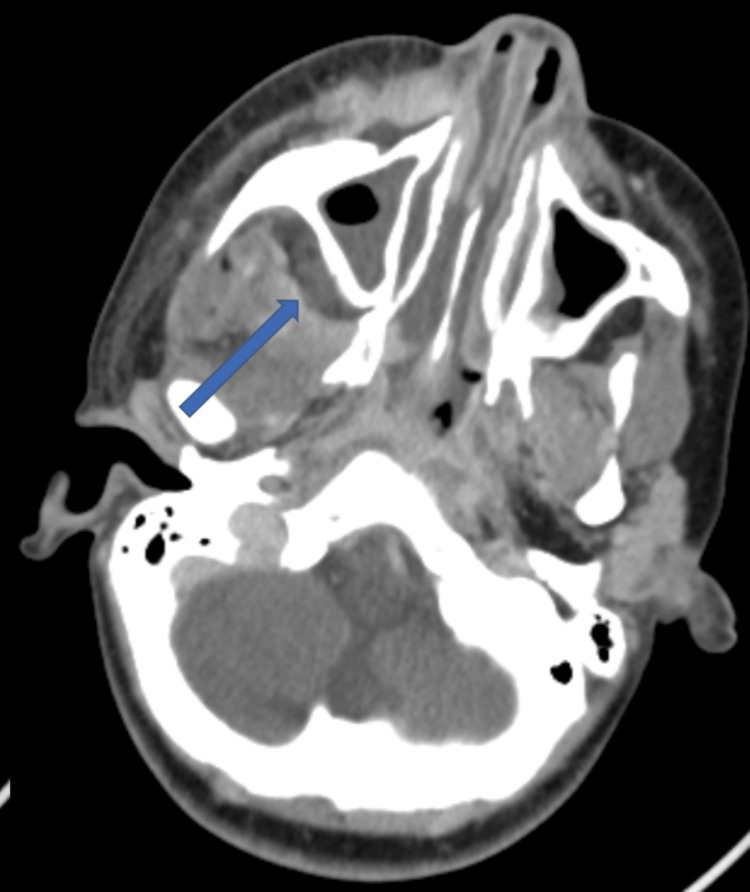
Axial CT image demonstrating opacification of the right maxillary and ethmoidal sinuses with extension of inflammatory infiltration toward the ipsilateral facial and orbital soft tissues (arrow) CT: computed tomography
